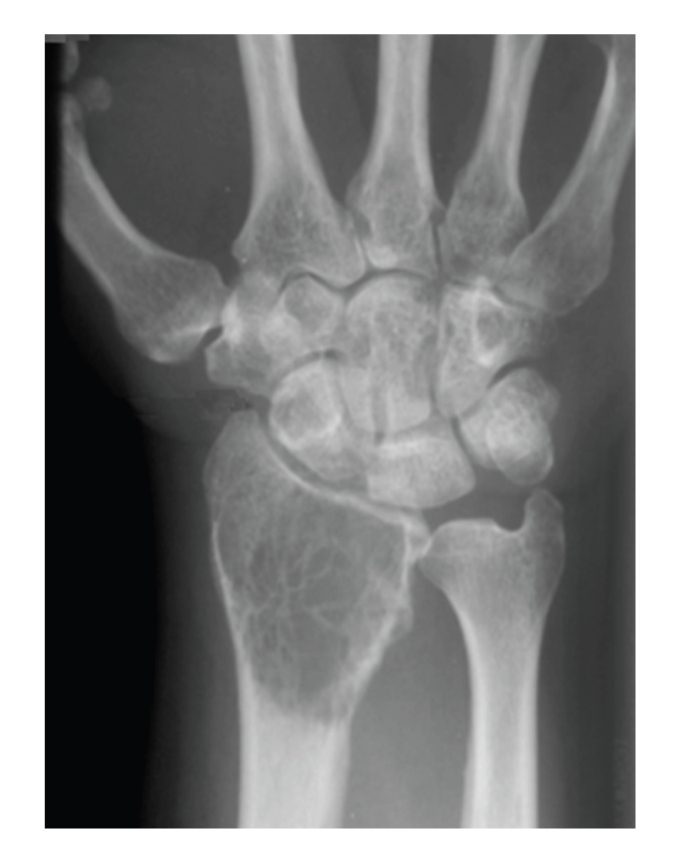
Preoperative radiographs demonstrating a giant cell tumour of the distal radius (seen as a classic lytic lesion).
